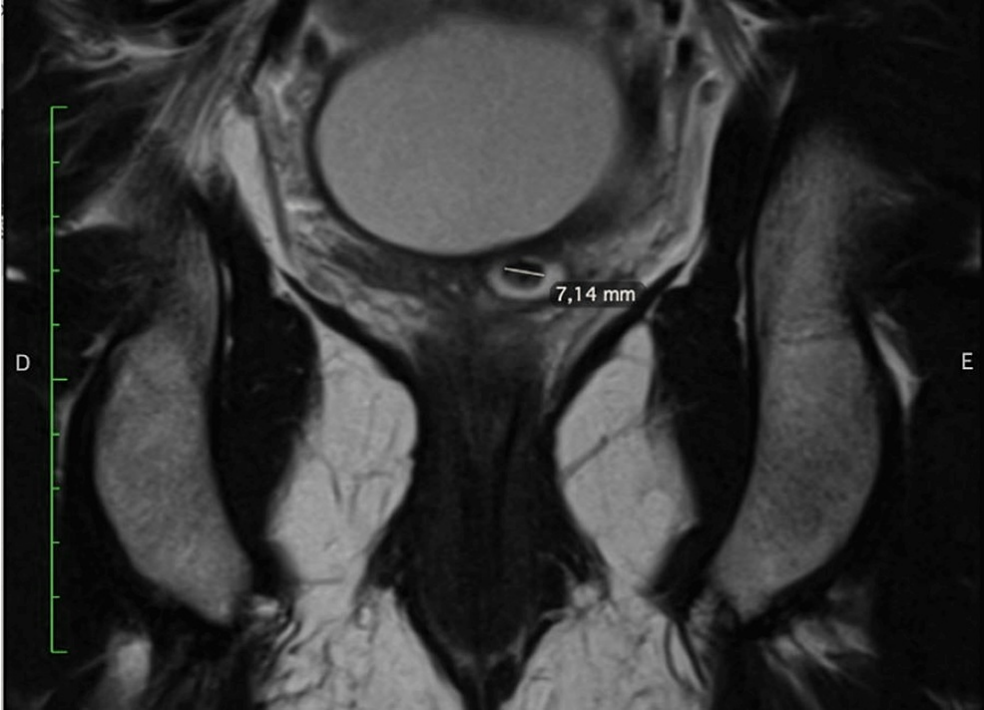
T2 MRI coronal view. MRI: magnetic resonance imaging
